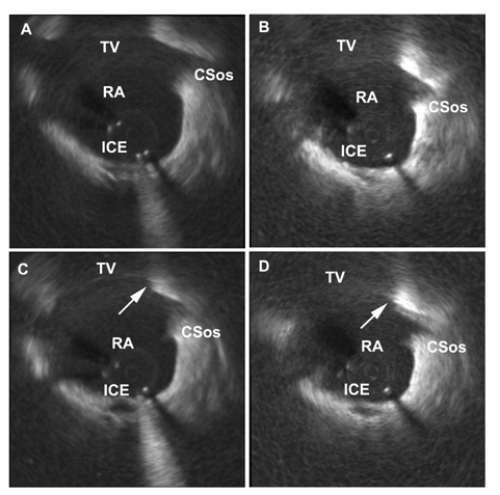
Two-dimensional intracardiac echocardiography images showing part of Koch's triangle between the tricuspid valve and the ostium of the coronary sinus under four different conditions. A: Native 2D horizontal cross-sectional echocardiography image before ablation. B: The same region before ablation with use of echocontrast. C: The same region after radiofrequency energy ablation without echocontrast infusion. A crater as an indirect sign of the ablation lesion (arrow) can be seen on the endocardial surface at the atrial side adjacent to the tricuspid valve. D: The same region after radiofrequency energy ablation and during echocontrast infusion. The ablation lesion (arrow) is visualized as a low contrast area within the atrial myocardial tissue. A crater can be seen on the atrial side adjacent to the tricuspid valve. In both C and D situations (post-ablation) there is significant swelling of the ablated region compared with pre-ablation situations (A and B). ICE = central artifact of the intracardiac echocardiography catheter, TV = tricuspid valve, RA = right atrium, CSos = ostium of the coronary sinus
